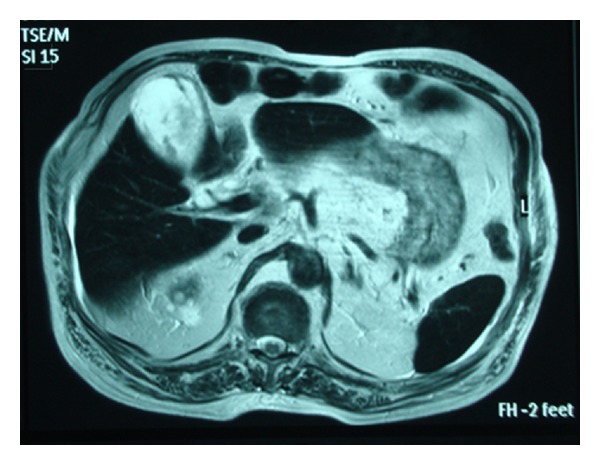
T2-weighted MRI showing a distended gallbladder enhanced by mixed signal intensity (hemorrhagic or exudative-like) component.
